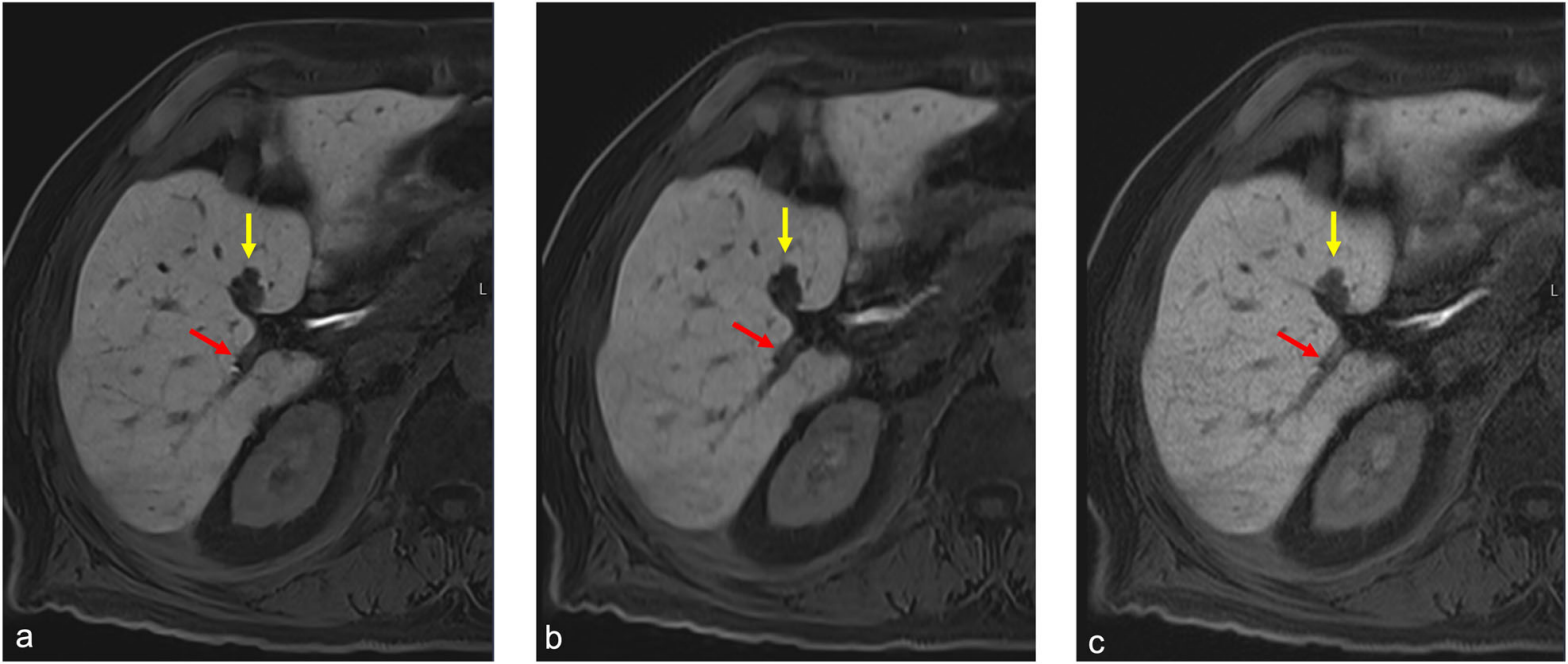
Axial T1-weighted water-only reconstructions from Dixon imaging in the hepatobiliary phase following administration of gadoxetate. Scores were higher for image quality, CNR, vessel edge sharpness (red arrow), and lesion edge sharpness (yellow arrow) for the 17-s (a) and 12-s (b) acquisitions using NN + ID compared with the 17-s acquisition with standard reconstruction (c)
